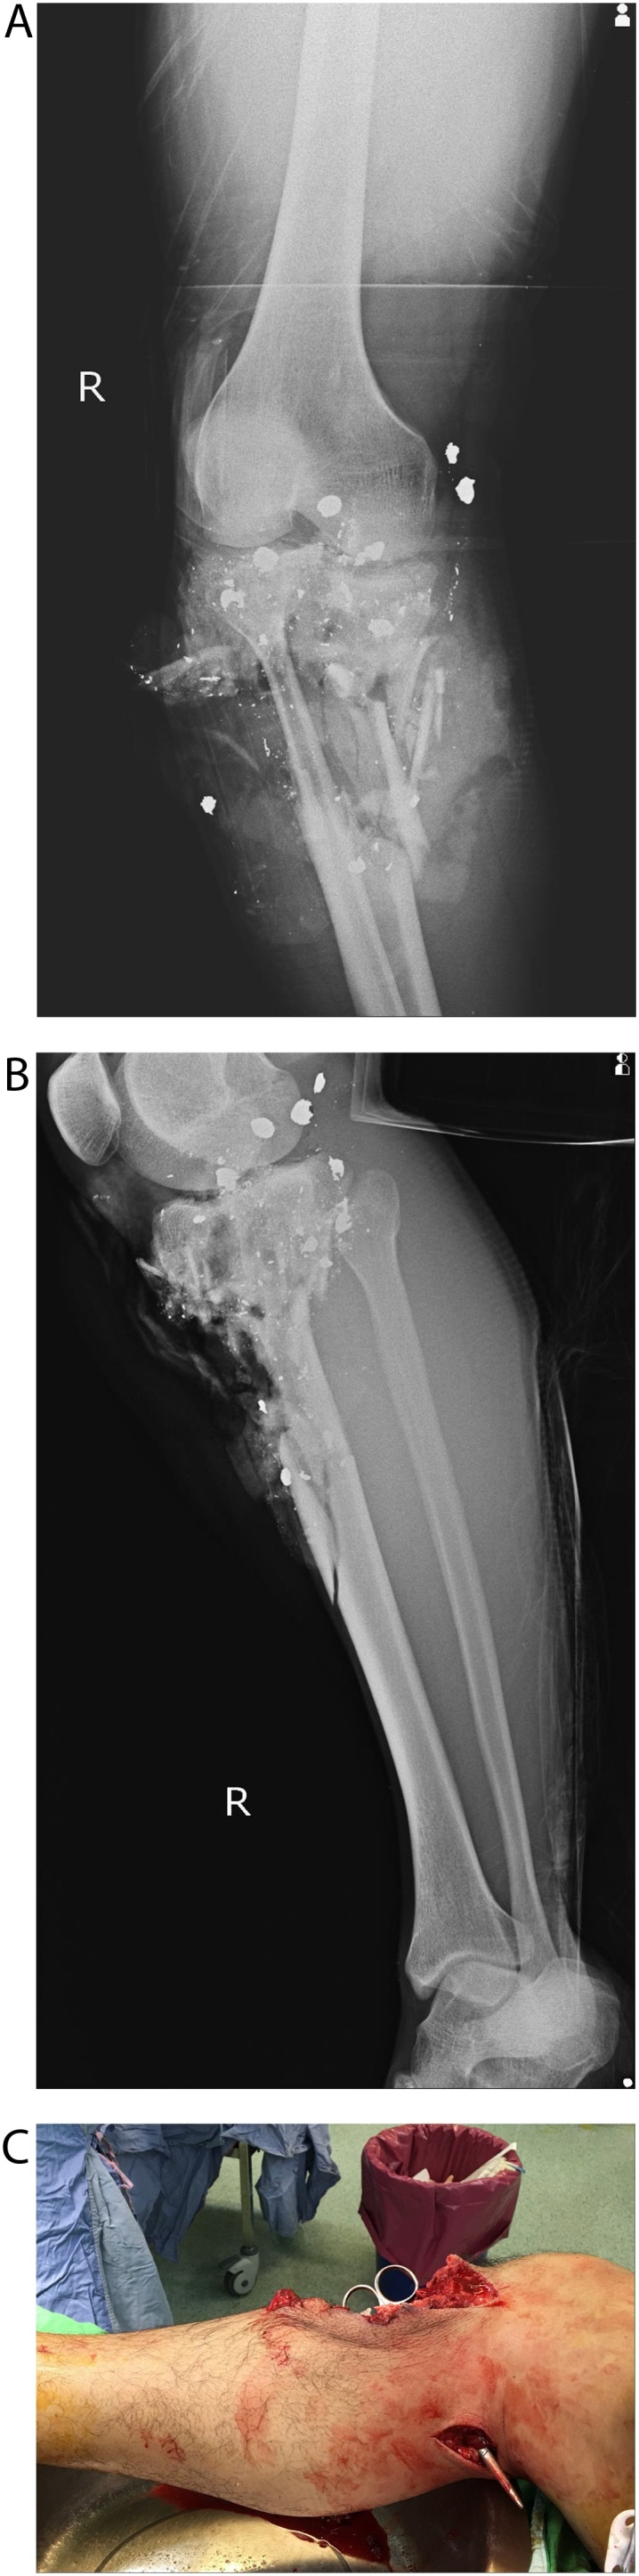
(A) Preoperative radiograph, AP view (B) Lateral view (C) Initial soft tissue loss caused by gunshot injury. The direction of the scissors indicates the bullet trajectory.
